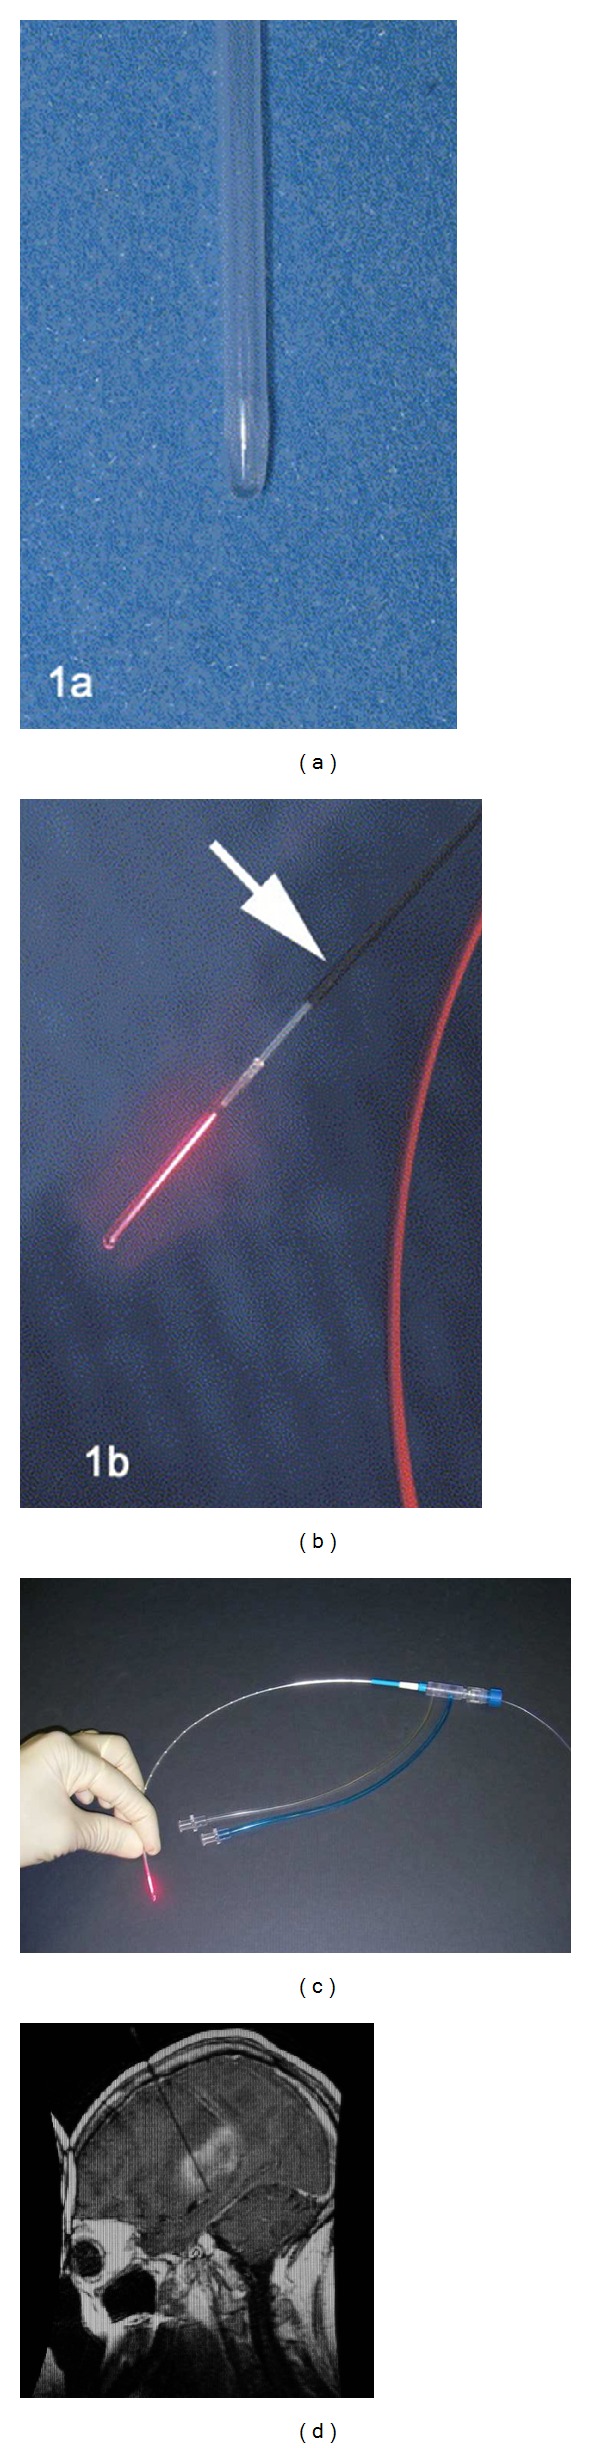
((a), (b)) Visualase applicator tip used in initial clinical investigation of LITT in GBM patients [4]. (a) Fiber protection sheath. (b) Illuminated fiber tip, arrow notes magnetite particle coating used for visual reference during MR imaging [4]. (c) Most recent iteration of Visualase laser applicator, shown with cooling catheter attachment. (d) MR reference image showing the insertion of the entire length of the applicator [9].
